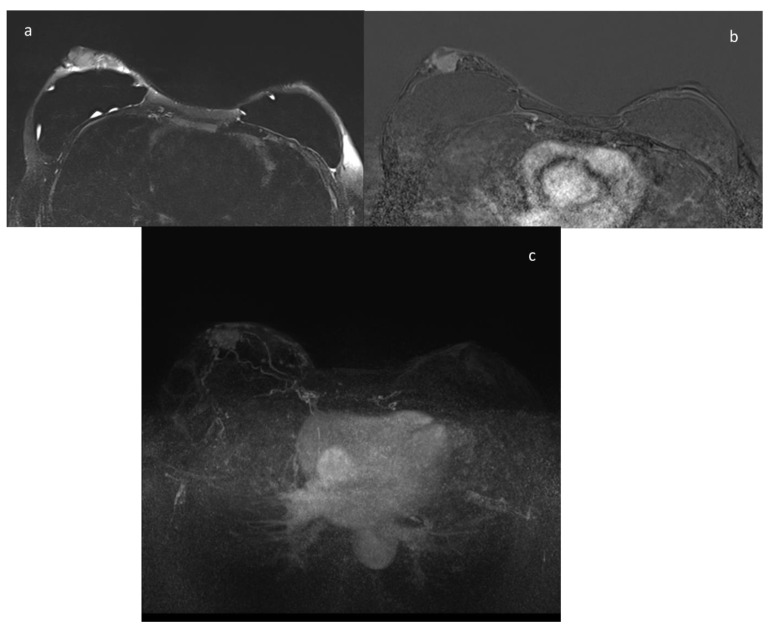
A 43-year-old patient with BRCA2 gene mutation; the patient underwent prophylactic mastectomy. At the annual ce-MRI screening a mass with irregular margins was identified in the retroareolar area of the right breast, corresponding at the US second look to a suspicious hypoechoic nodule that undergoes core needle biopsy with the diagnosis of invasive lobular carcinoma. (a) T2-weighted imagine; (b) contrast-enhanced image; (c) MIP image.
